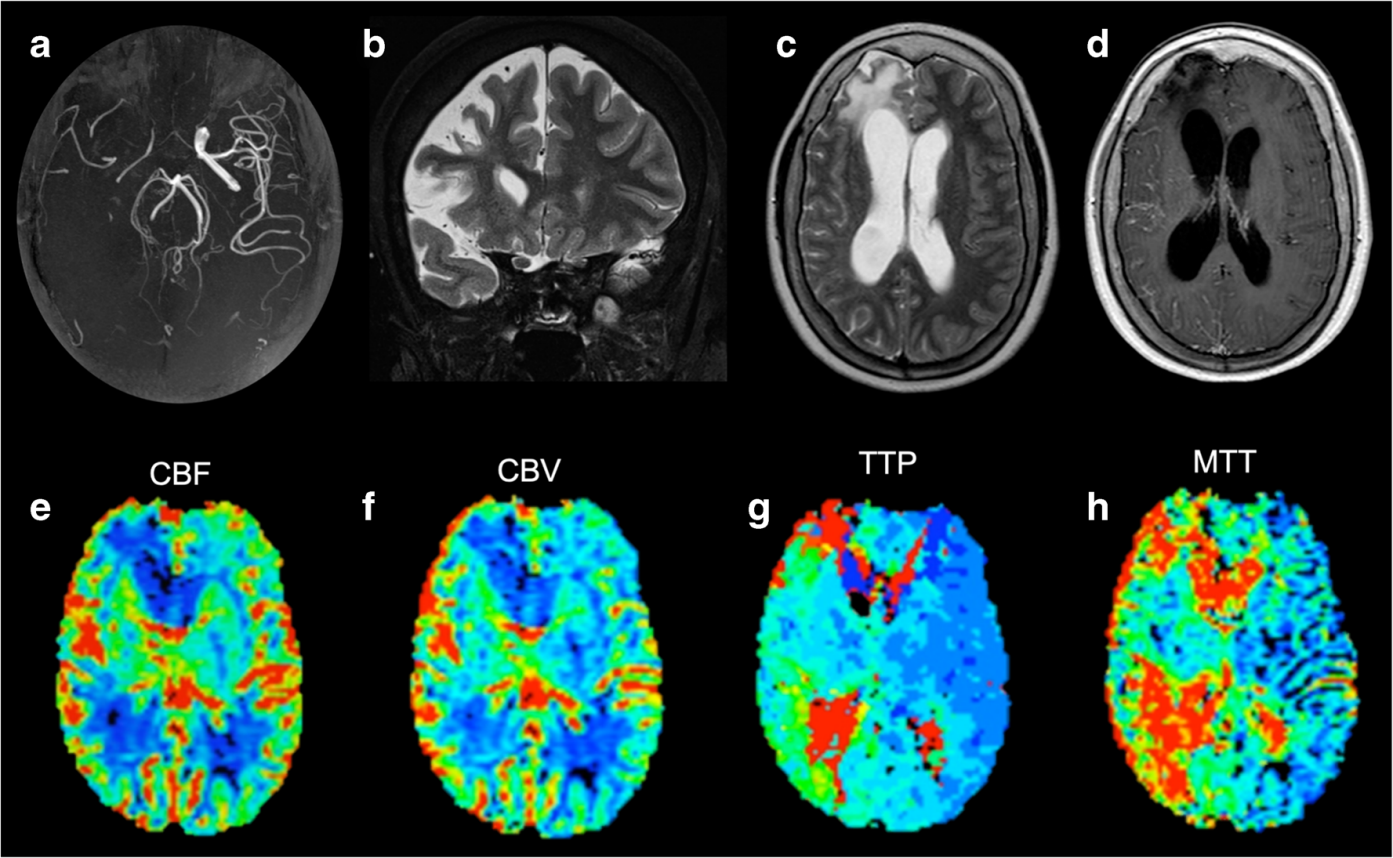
Imaging findings in patient #9. Occlusion of the right intracranial ICA is evident on MRA (a). The right frontal lobe appears shrunken and hyperintense on T2-weighted images (b–c), due to the chronic hypoperfusion. Contrast-enhanced T1-weighted sequence shows ipsilateral leptomeningeal enhancement and MM vessels (d). Reduction of CBF (e) and CBV (f) of the right atrophic frontal lobe was observed, with increase of the whole right hemisphere on TTP (g) and MTT (h) maps. Please note the marked signal increase on the same maps among the remaining right hemisphere
